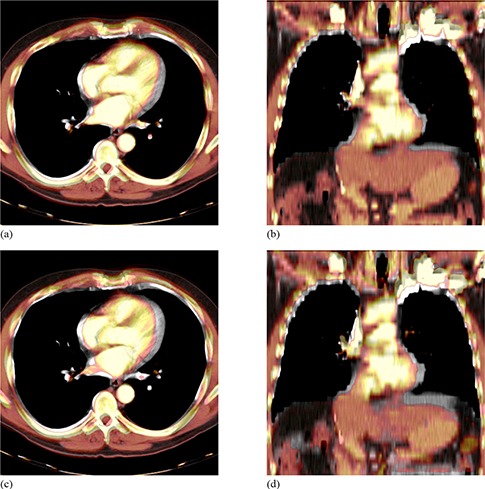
Sample colored warped images overlaid on greyscale baseline images depicting the range of magnitudes of synthetic B‐spline warped images used for validation of thoracic CT registrations: axial (a) and coronal (b) views of images that differ by a displacement error of 4.6 mm and an axial displacement error of 3.4 mm; axial (c) and coronal (d) views of images that differ by a displacement error of 9.4 mm and an axial displacement error of 7.1 mm.
